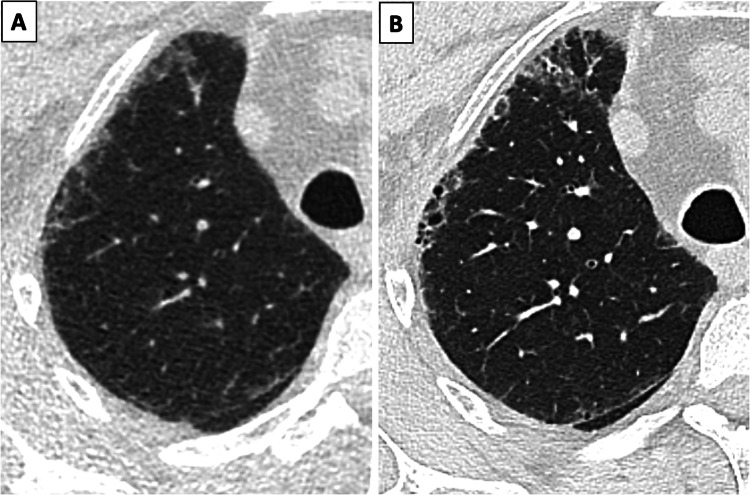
Example of ILA detected on baseline LCS low dose computed tomography (A), with evidence of progression toward over fibrosis at standard dose chest CT (B) performed after 36 months
